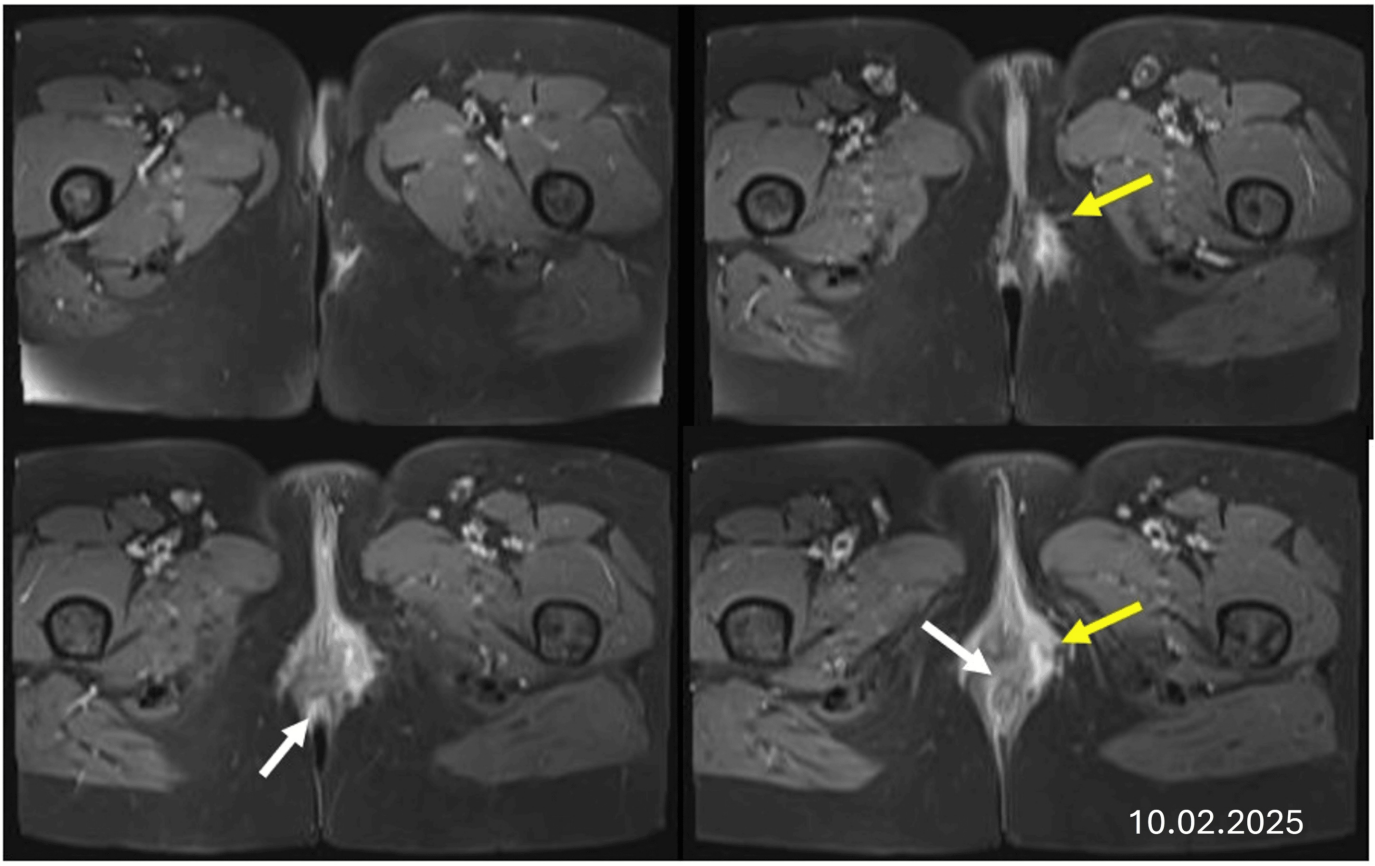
Axial T2-weighted fat-suppressed images demonstrate the recurrent anal fistula after the patient’s third surgery. The white arrows indicate the internal opening at the anal canal, and the yellow arrows mark the external opening in the left anterior perineal skin.
